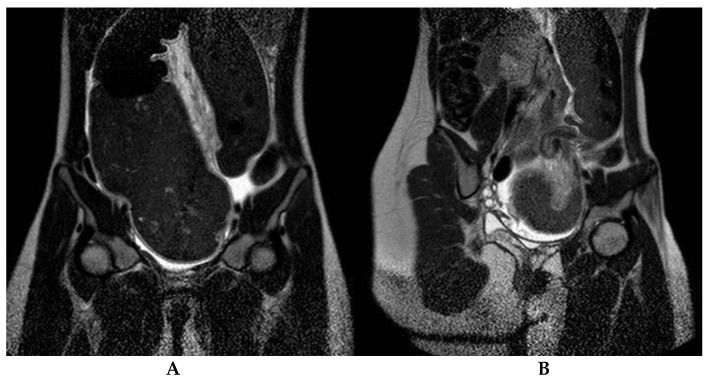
Sigmoid colon volvulus in a 14-year-old girl. Coronal T2 weighted MRI images demonstrate closed-loop obstruction of the sigmoid colon with severe visceral dilatation (A) and whirlpool sign (B); abdominal free fluid is also depicted.
